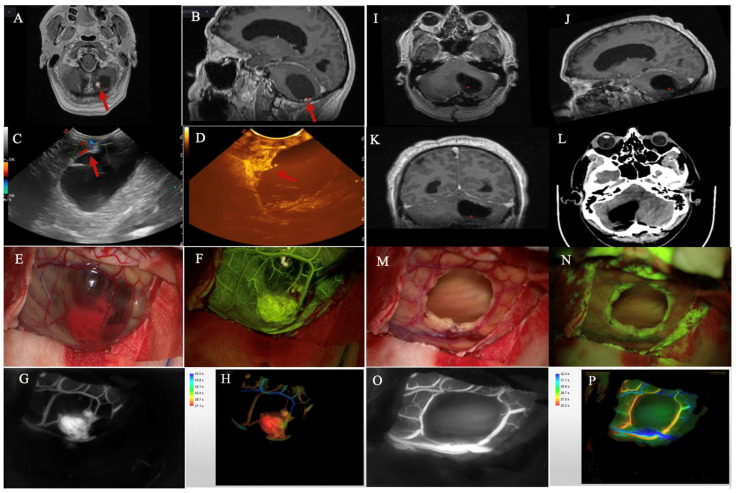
Cerebellar HB. (A,B) Preoperative axial and sagittal T1 after contrast medium administration MRI scans showing a right cerebellar HB, characterized by an intramural nodule (red arrow) and a cystic component. (C,D) IOUS and iCEUS images obtained after dural opening show the lesion’s cystic component, characterized by early enhancement of the intramural nodule (red arrow). (E) Intraoperative view of the lesion under white light. (F) Intraoperative view of the lesion after a bolus-type injection of SF to perform a SF-VA, which highlights the nodule and peritumoral cortical vessels. (G,H) Intraoperative vision after ICG-VA and FLOW800 analysis, evaluating perilesional hemodynamics. (I–L) Postoperative axial, sagittal, and coronal T1 after contrast administration MRI (I,J) and axial CT images (L) showing complete removal of the intramural nodule. (M,N) Intraoperative vision after lesion removal under white light and YELLOW560 filter. (O,P) Intraoperative control ICG-VA and FLOW800 showing preserved perilesional vessels with their quantitative blood flow evaluation.
